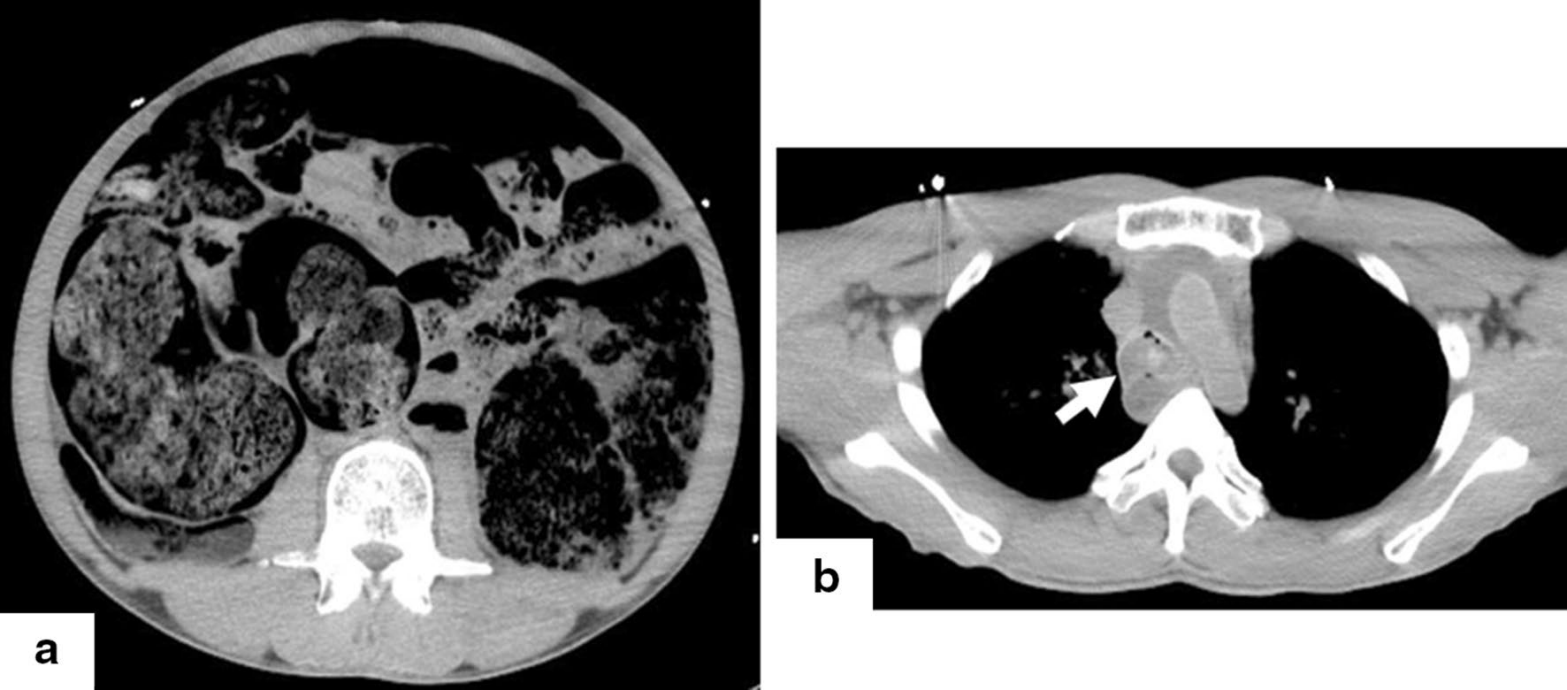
Postmortem computed tomography (PMCT) of a 40-year-old man with mental retardation. a Abdominal CT showing a distended small intestine and colon with an intraluminal heterogenous mass. b The trachea was filled with residue (*arrow*).
